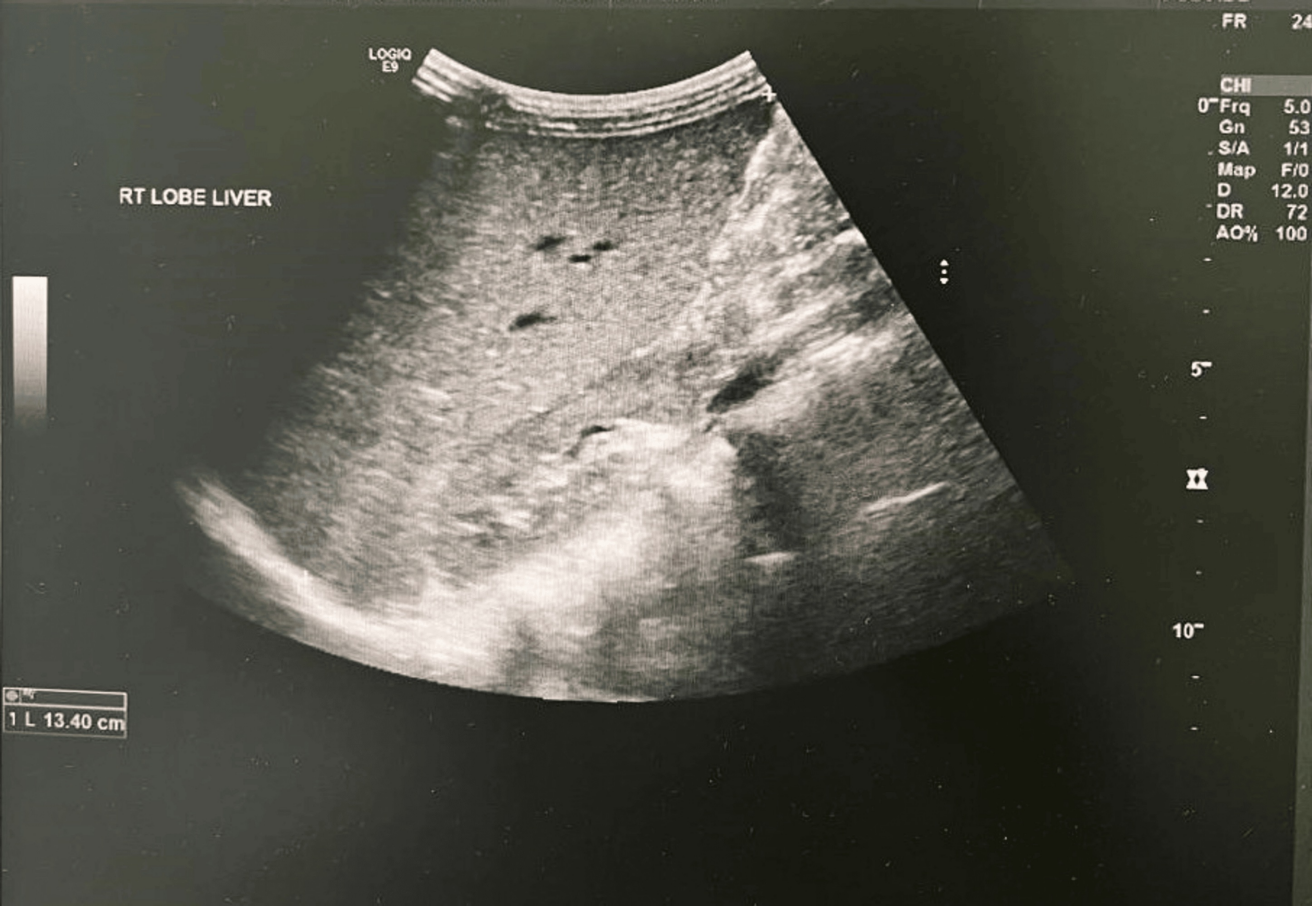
Ultrasound showing liver size 134mm
